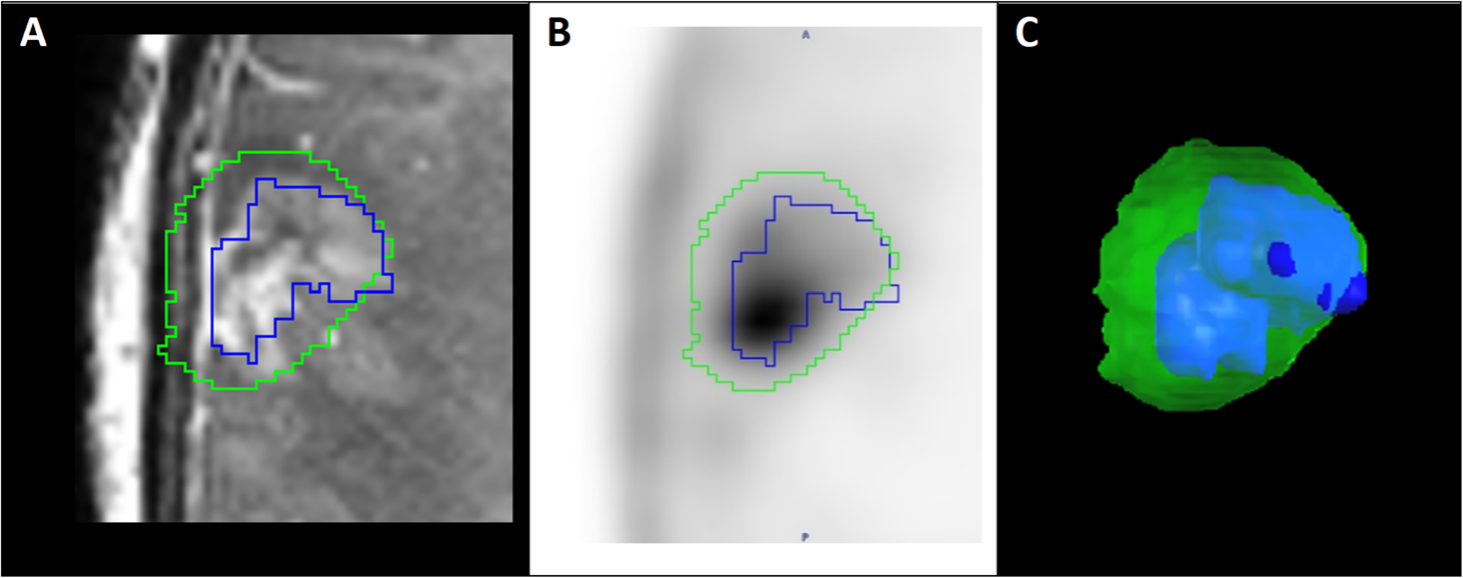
PET and MRI VOIs of cerebral tumour from scan 4. An example of the relationship between PET (green) and MRI (blue) tumour volumes that occurred in all four scans. a Zoomed in axial view of T1 MRI_CE_ image and b PET image from scan 4. c 3D representation showing PET VOI encompassing MRI_CE_ VOI from scan 4
